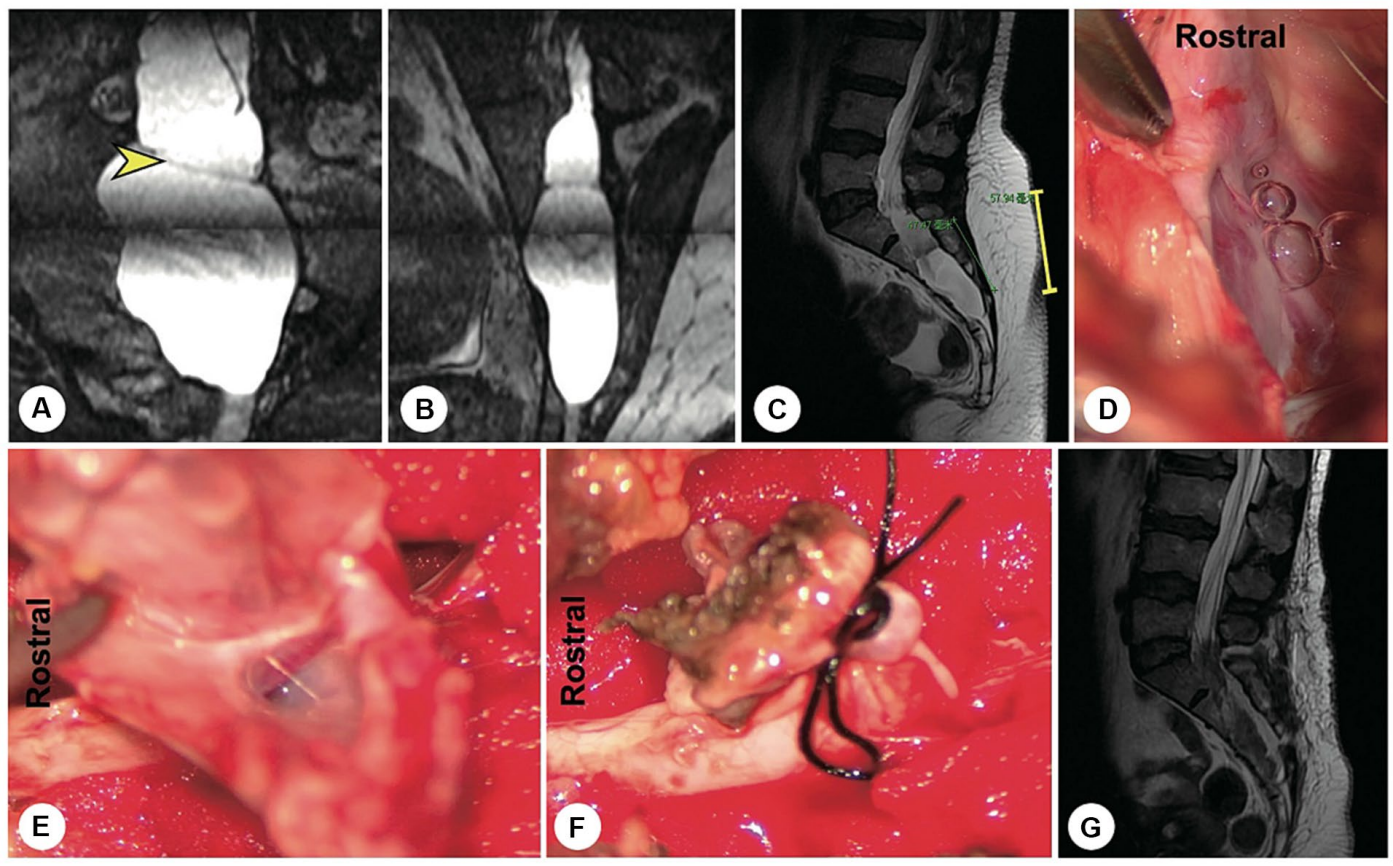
A representative patient with a fistula-type cyst. (A,B) Preoperatively, reconstructed MR image showing a fistula between the dural sac and the cyst (yellow arrowhead). (C) A 5 cm precise minimally invasive incision was designed (yellow line segment). (D) Intraoperatively, a fistula-type cyst was found, and there was no nerve root. (E) The cyst wall was dissected and turned over. (F) The cyst neck was ligated. (G) Follow-up MRI showed that the cyst had disappeared and that there was no recurrence.
